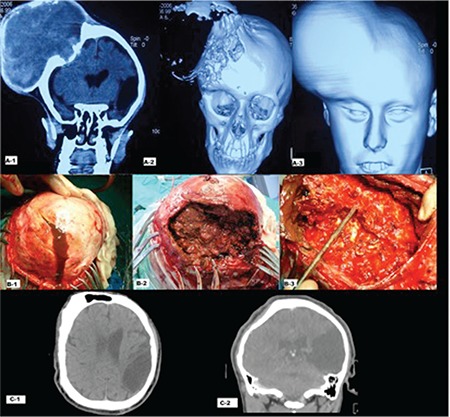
A-1, A-2, A-3: Multidetector computed tomography scan with reconstruction shows large lytic intradiploic lesion with expansion and scalloping of the bony margins; please note that the inner and outer tables are separated and destructed. B-1, B-2, B-3: Intraoperative images; evacuated lesion was mud-like, inner table was protected. C1, C2: Sagittal and coronal computed tomography images after 7 years; good and acceptable remodeling of the calvarium is seen.
